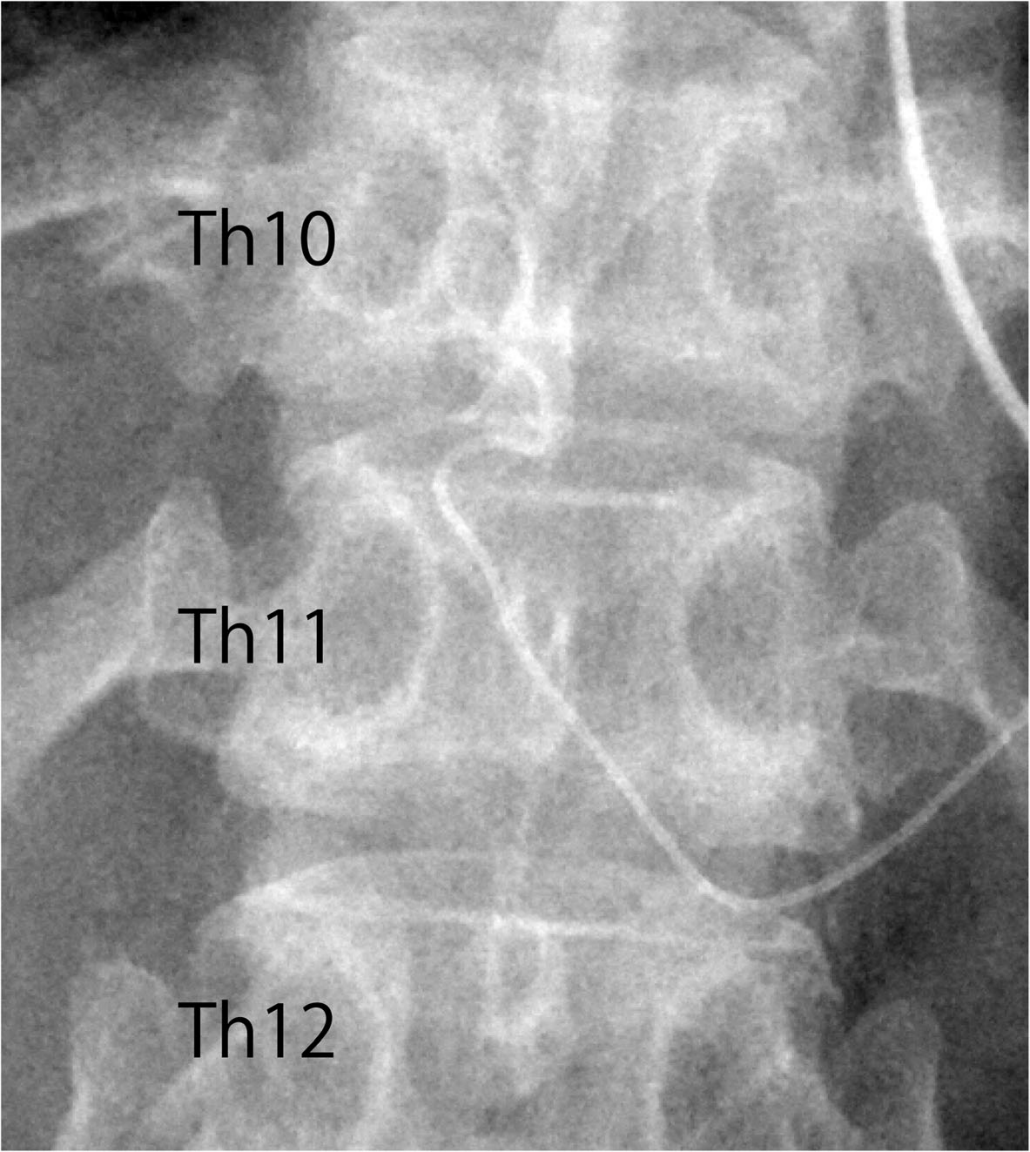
A plain abdominal X-ray taken after the surgery
